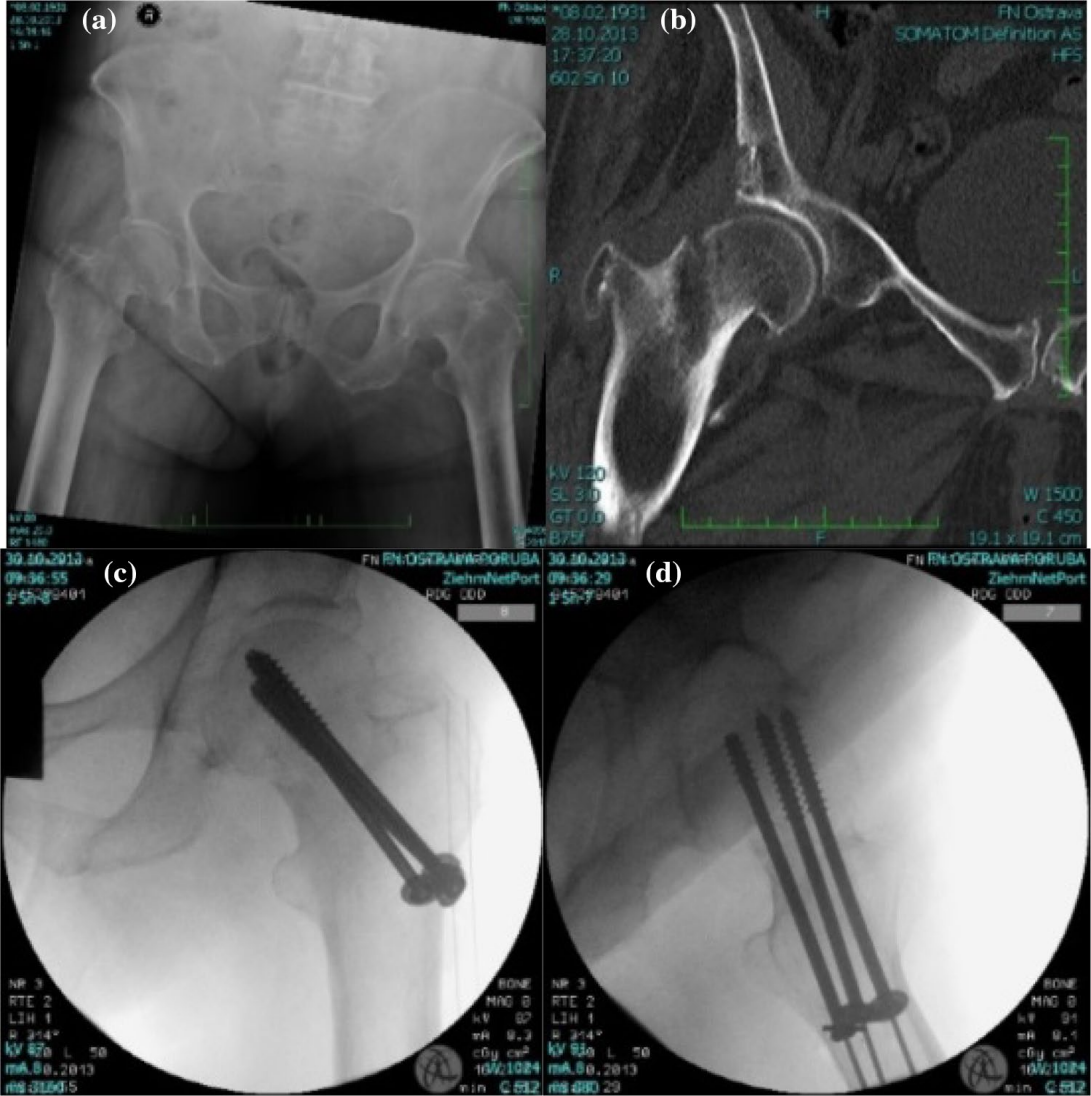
Femoral intracapsular fracture a pelvic X-ray, b CT scan, c Osteosynthesis with three lag spongious screws, d Osteosynthesis with three lag spongious screws
